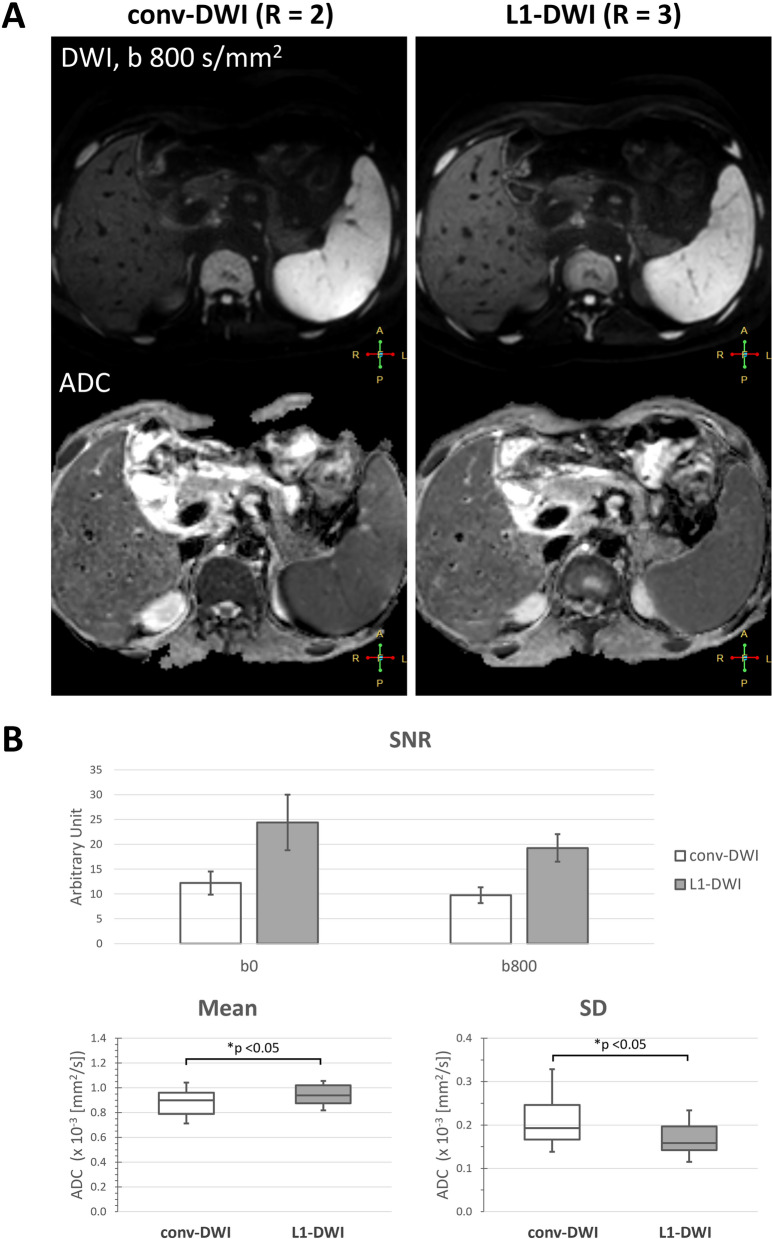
Comparison between liver conv-DWI (left) and L1-DWI (right) in healthy volunteers. conv-DWI = single-shot EPI-based DWI with parallel imaging reconstruction. L1-DWI = single-shot EPI-based DWI with L1-regularized iterative reconstruction. SD = standard deviation. (A) Images of a 35-year old volunteer of DWI at b = 800 s/mm^2^, and the resulting ADC image. (B) Higher SNR in DWI for both b = 0 and 800 s/mm^2^, as well as less variable ADC values were observed in L1-DWI in comparison to conv-DWI in 12 volunteers.
